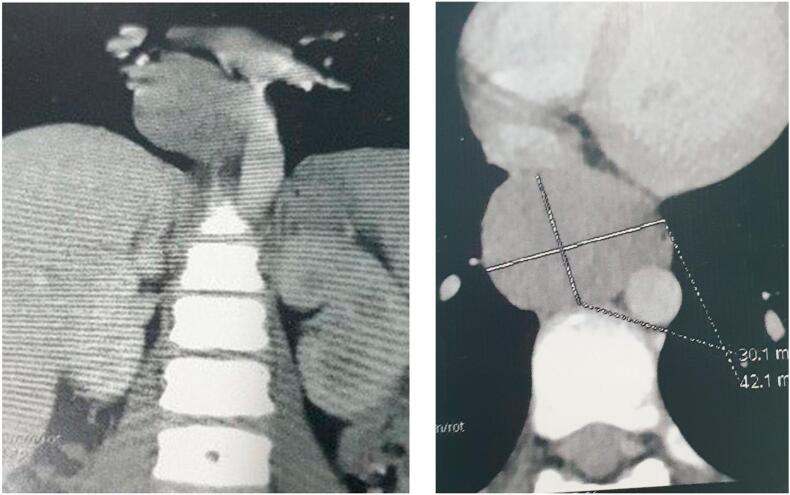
Chest CT scan showing cystic formation of the middle mediastinum and right lower esophagus.
